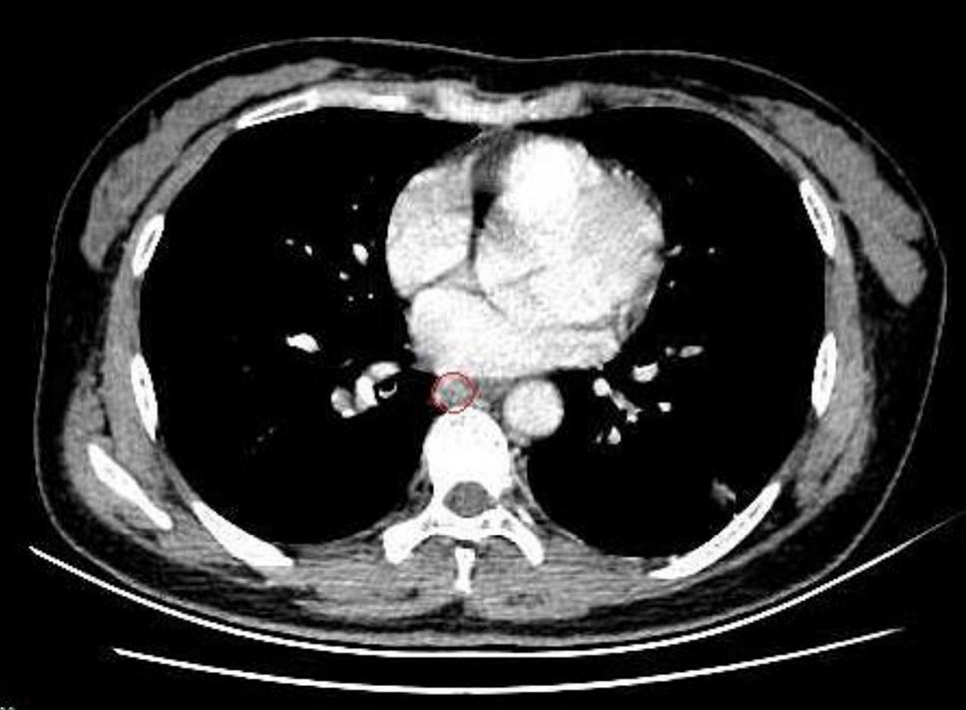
Chest CT scan revealed a sharply marginated mass, 2 × 3 cm in size, in the posterior mediastinum (marked in red circle)
